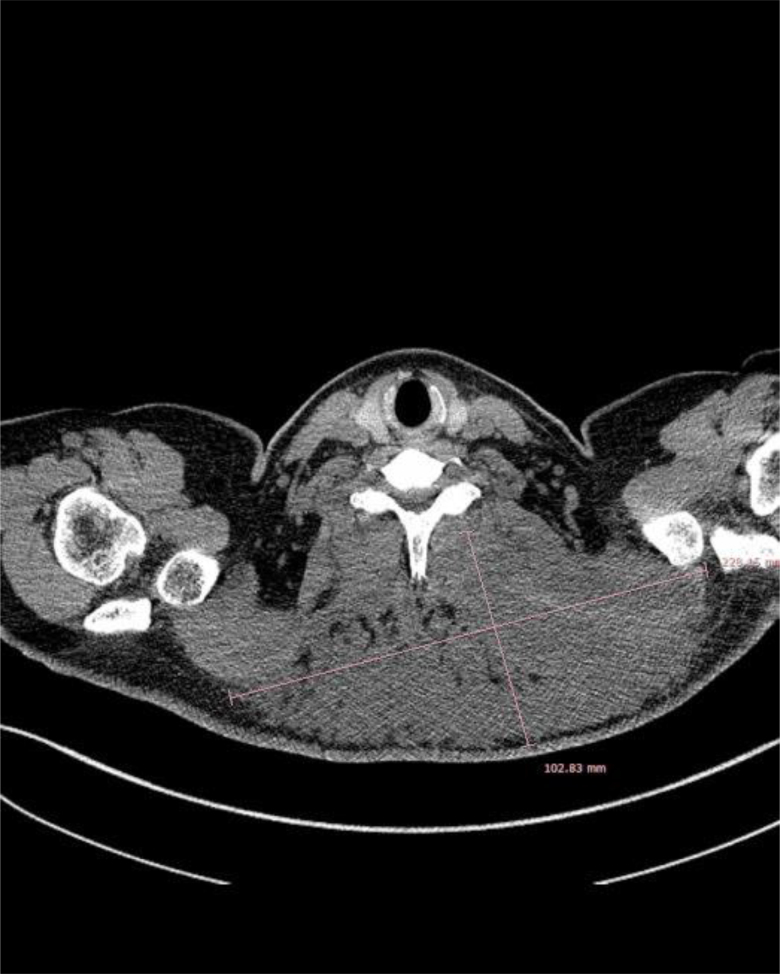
Initial chest computed tomography scan. The initial chest computed tomography scan of the patient’s back reveals a lesion measuring approximately 10.2 × 22.8 cm. A large cystic lesion surrounded by extensive soft-tissue infiltration is observed in the upper back. Additionally, the examination indicates involvement of the back muscle by the lesion.
